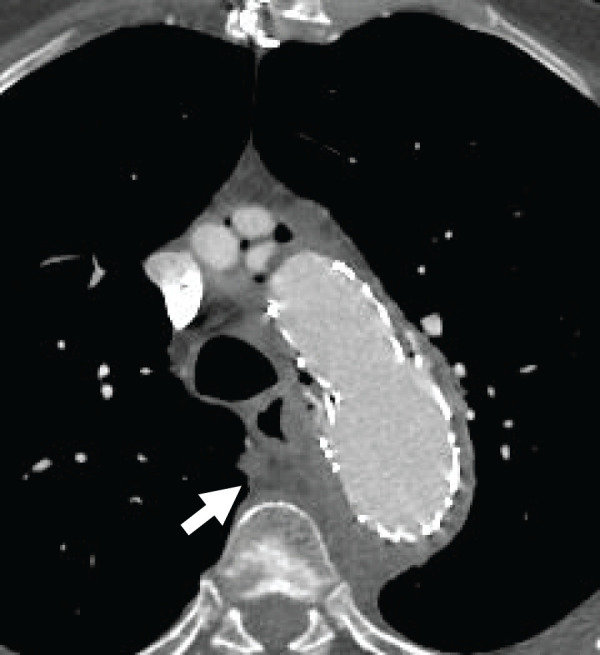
The CT results revealed free air surrounding the prosthetic graft and the collection of fluid resembling an abscess around the esophagus (white arrow).
